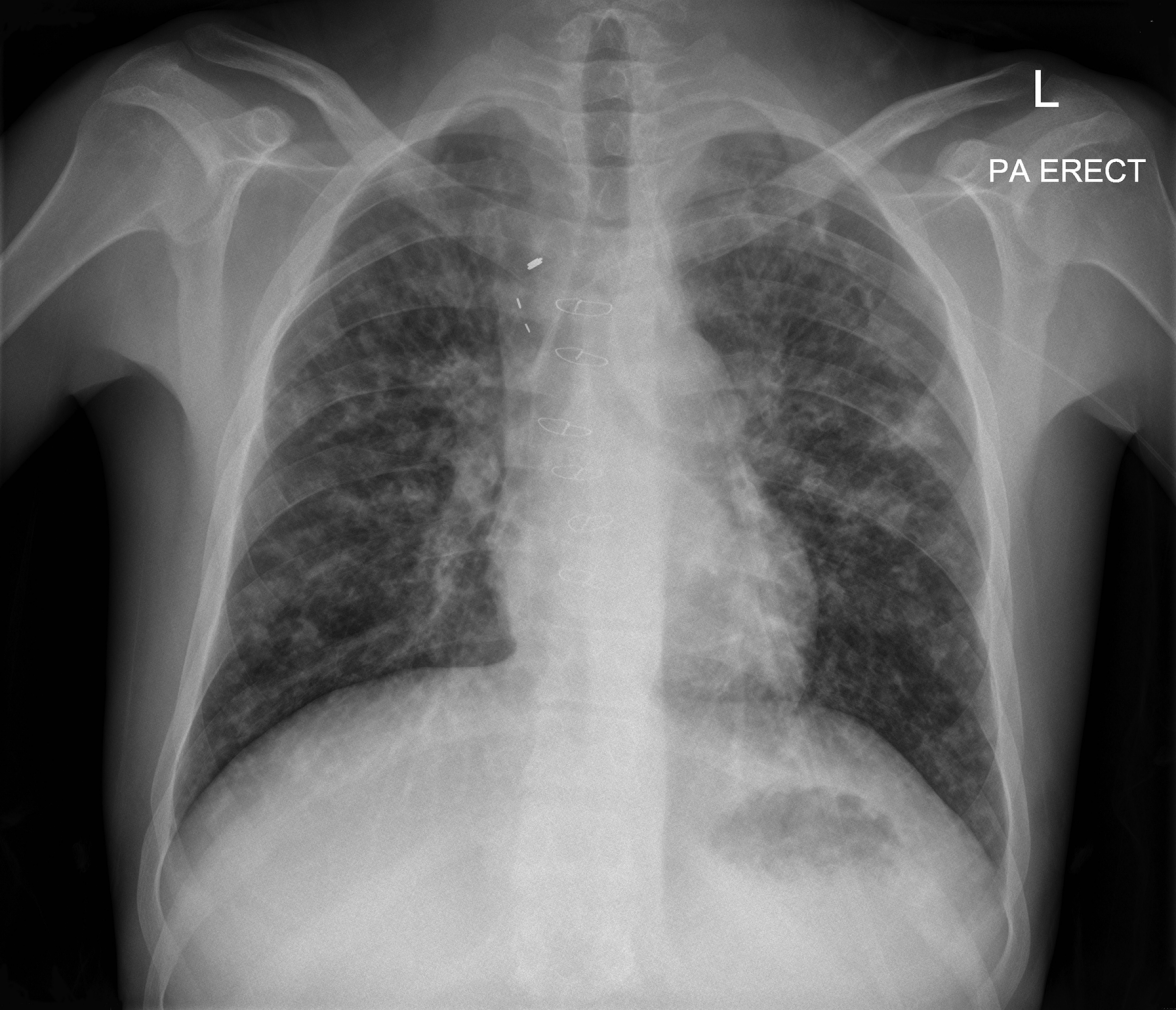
Chest radiography on admission demonstrating bilateral patchy consolidation on a background of bronchiectatic changes, with midline sternotomy wires and mediastinal clips from previous Fontan surgery.
